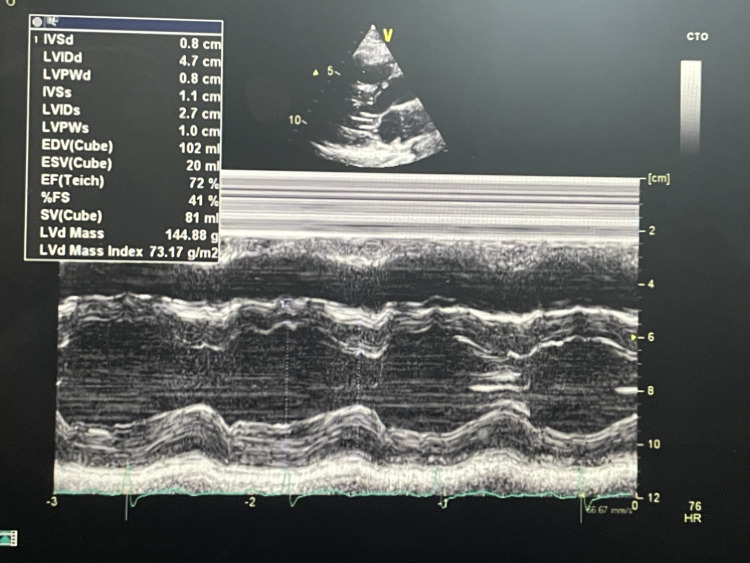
M-mode echocardiogram demonstrating normal left ventricular dimensions and normal function with ejection fraction of 72%.
